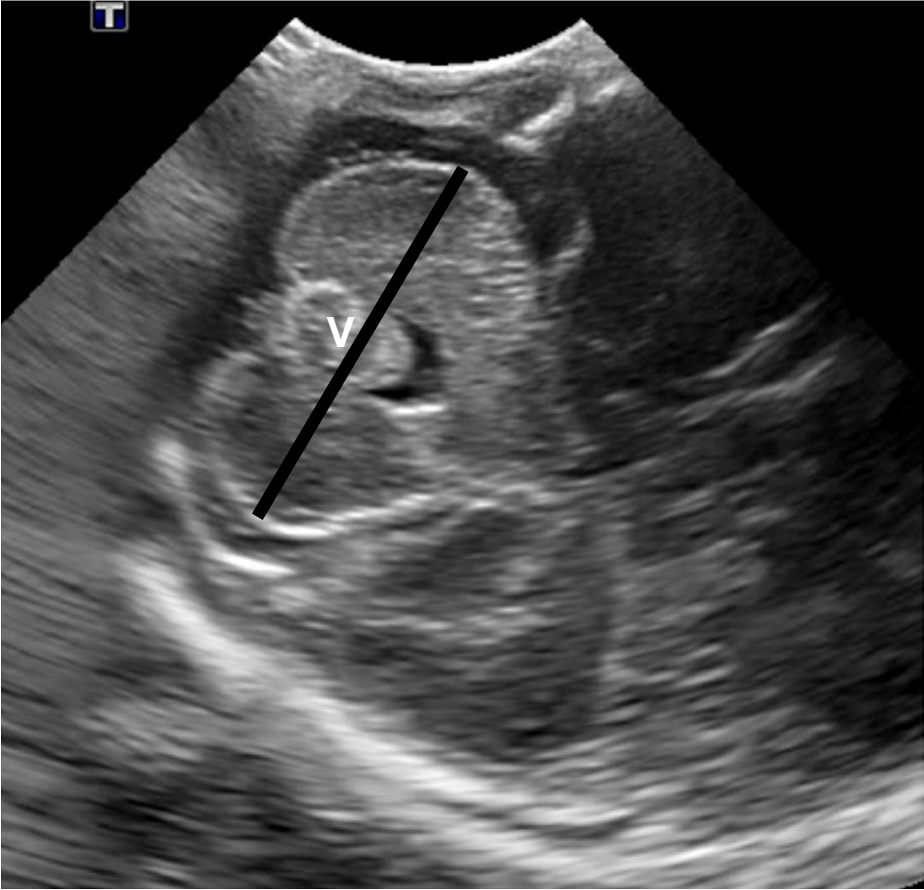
Transmastoid ultrasound image of a 7-day-old male born at 35 weeks of gestation showing symmetrical cerebellar hemispheres. The *black line* demonstrates the greatest width from the lateral aspect of one cerebellar hemisphere to the other side. “*V*” depicts the cerebellar vermis
